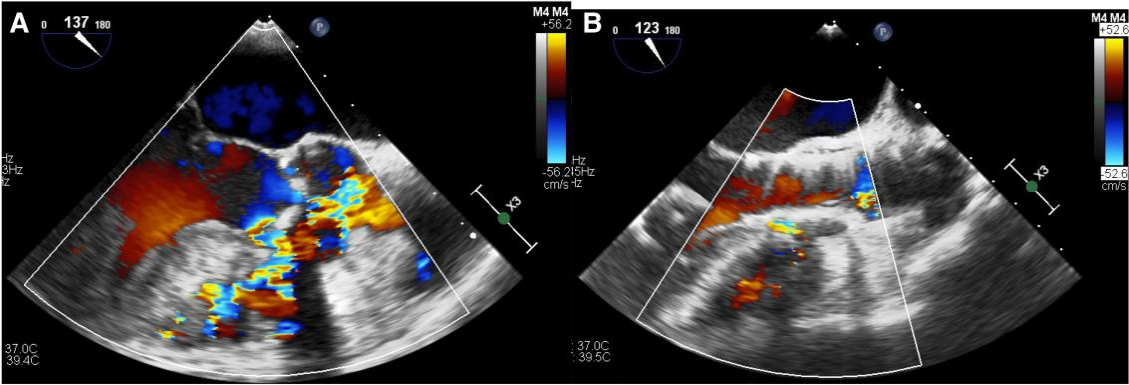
(*A*) Trans-oesophageal echocardiogram before trans-catheter aortic valve replacement revealed left-to-right shunt. (*B*) Trans-oesophageal echocardiogram after trans-catheter aortic valve replacement revealed the complete coverage of ventricular septal defect orifice by the valve and decreased left-to-right shunt without structural change of the left ventricular outflow tract and the left ventricle.
